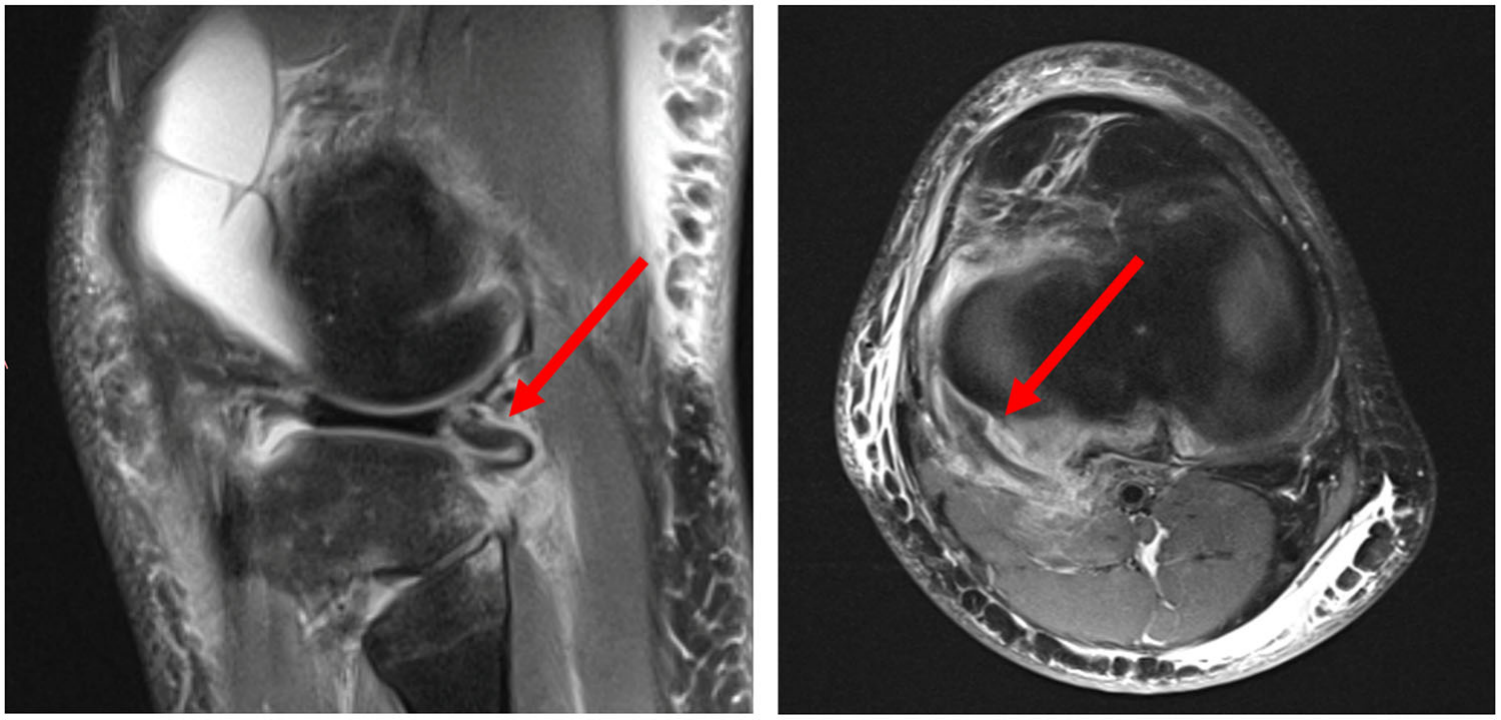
Sagittal (left) and axial (right) MRI scan with a ruptured popliteus tendon (red arrow). MRI, magnetic resonance imaging.
